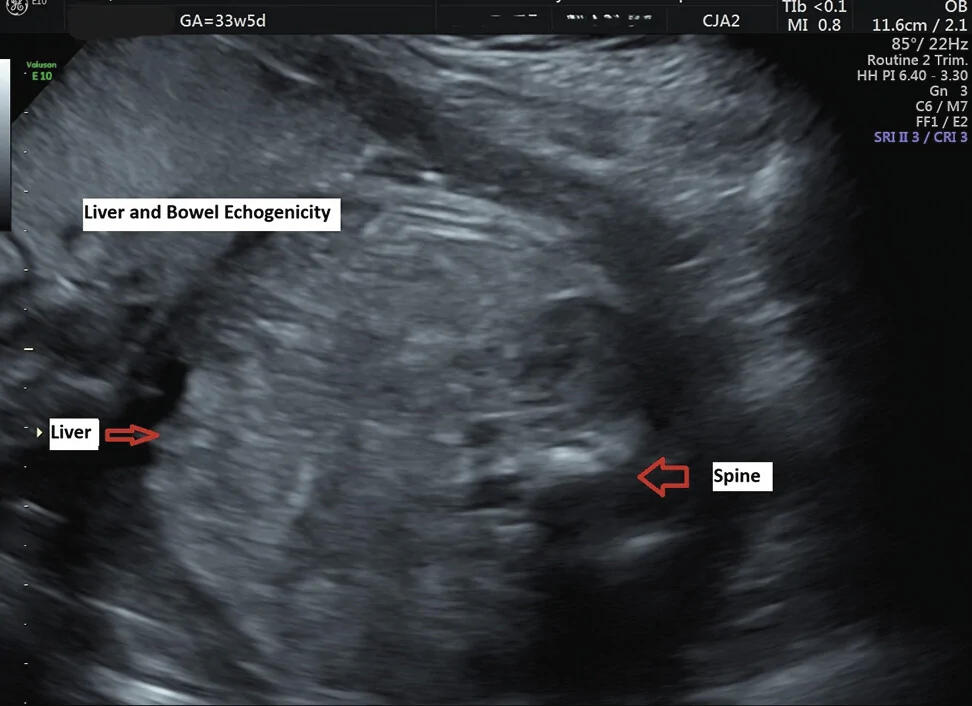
Echogenic bowel and liver. Transverse sonogram through fetal abdomen at 33 weeks of gestation. Increased echogenicity seen in the liver and also bowel as bright as the bone of the fetal spine in several areas. Arrows point to echogenicities in the fetal liver, and the spine is shown as a reference.
